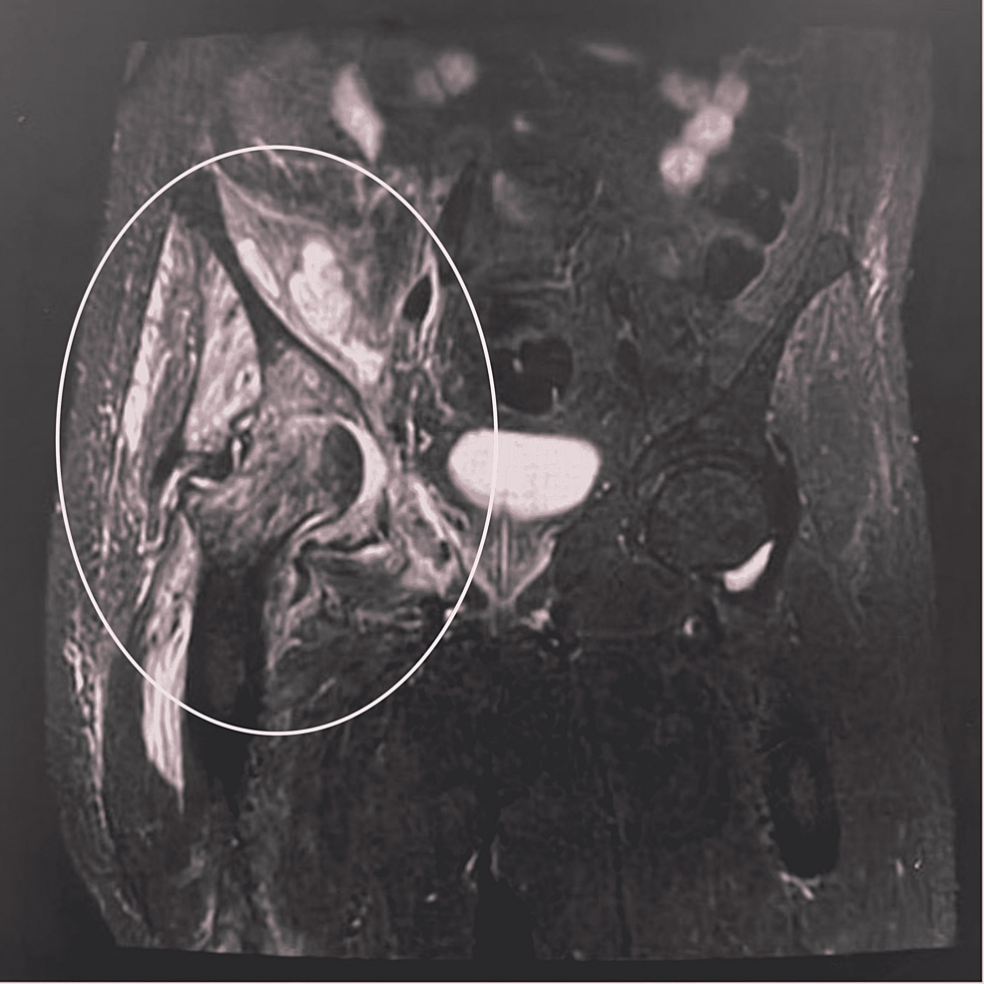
Pelvic MRI showed right hip septic arthritis and large iliacus muscle abscess.
